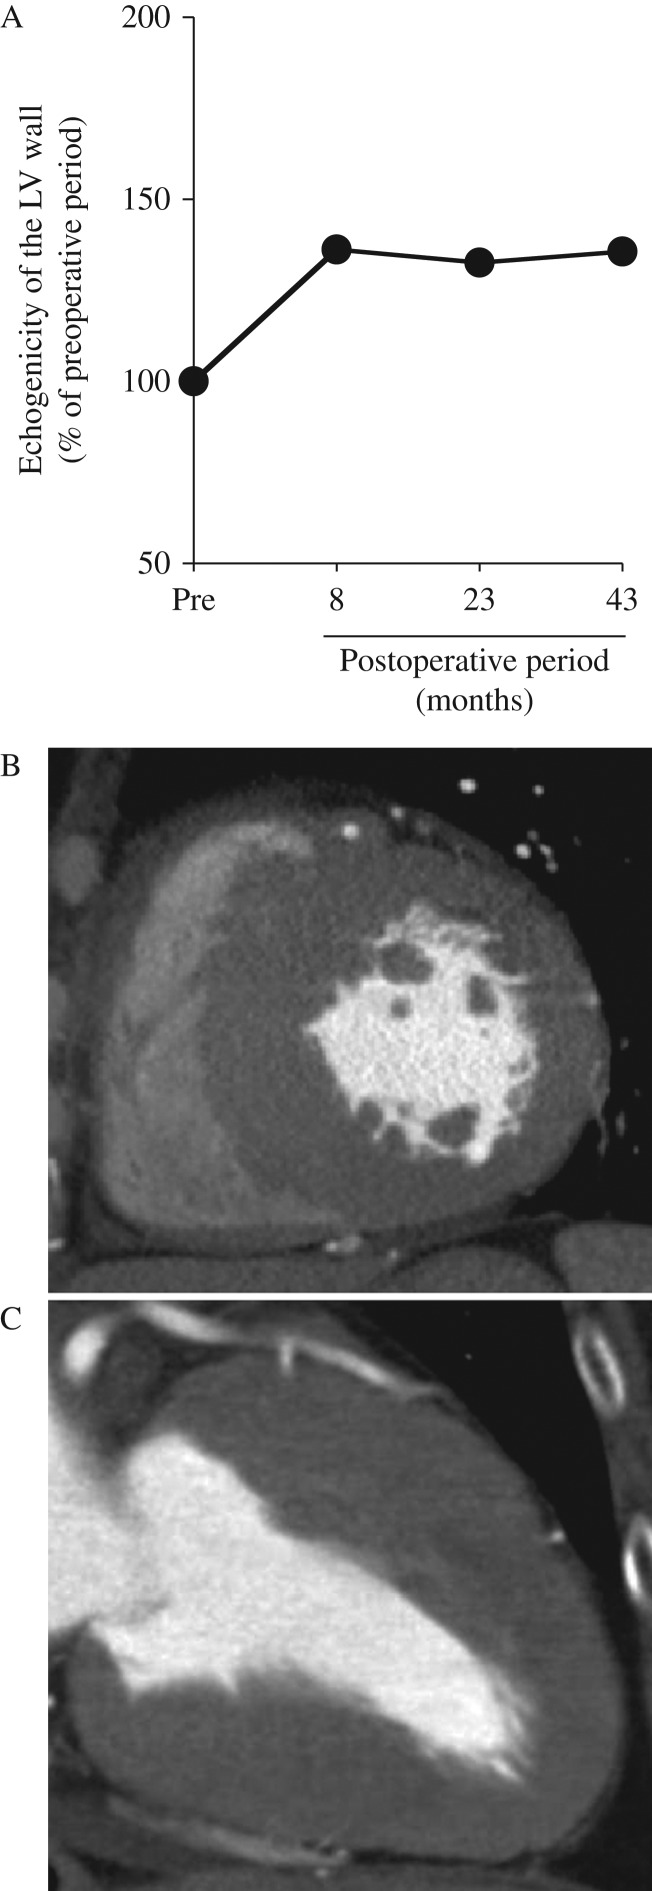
Change in ultrasound reflectivity and characteristics of CT signal density of the left ventricular wall. A 9×9 mm region of interest imaged by echocardiography was positioned in the mid-myocardium of the anteroseptal and posterior walls, and a 2×2 mm region of interest was positioned in the pericardium for reference. The ultrasound reflectivity of the regions was quantified using the NIH Image Software (Image J version 1.2; National Institutes of Health, Bethesda, MD, USA). The values in the graph (closed circles) represent the relative levels of echogenicity of the anteroseptal and posterior walls to those of the pericardium (A). Short-axis (B) and axial images (C) by contrast-enhanced cardiac multidetector-row CT showed myocardial hypoenhancement in the anteroseptal wall before the treatment.
